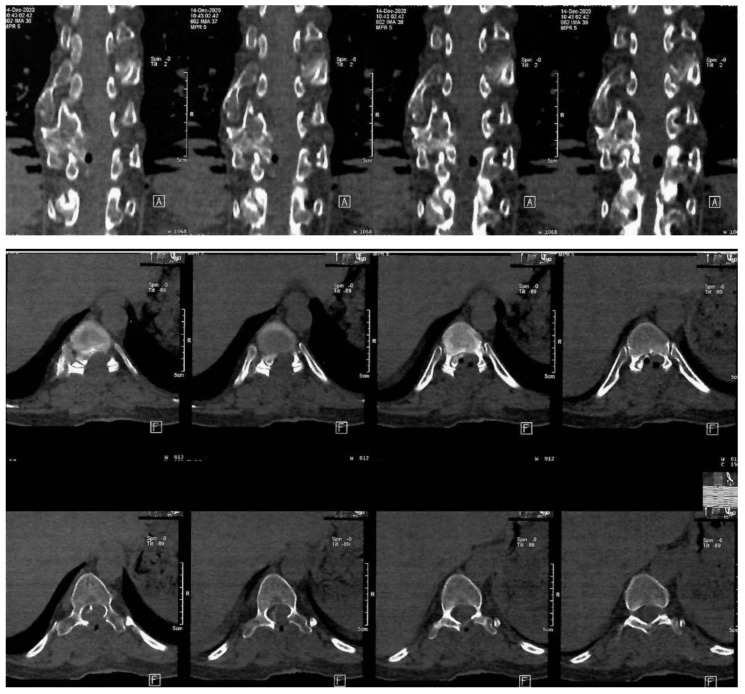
Postoperative thoraco-lumbar native CT scan. A: indicate the anterior (front) side of the body relative to the position of the cross-sectional slices being viewed, F: indicates the orientation of the patient’s feet during the scan.
